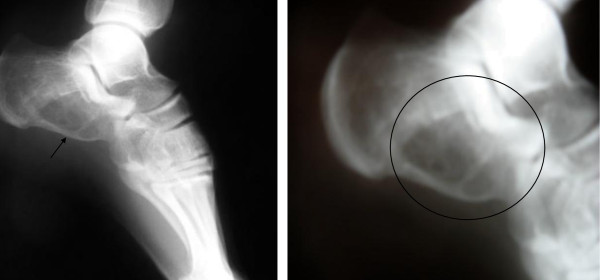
Radiograph showing an expansive but well-marginated osteolytic lesion in the front portion of the patient's left heel.
